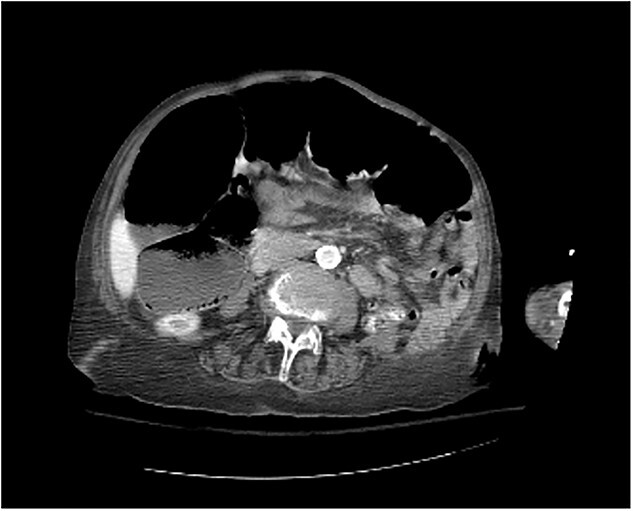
Colonic dilation seen on CT abdomen and pelvis with contrast.
